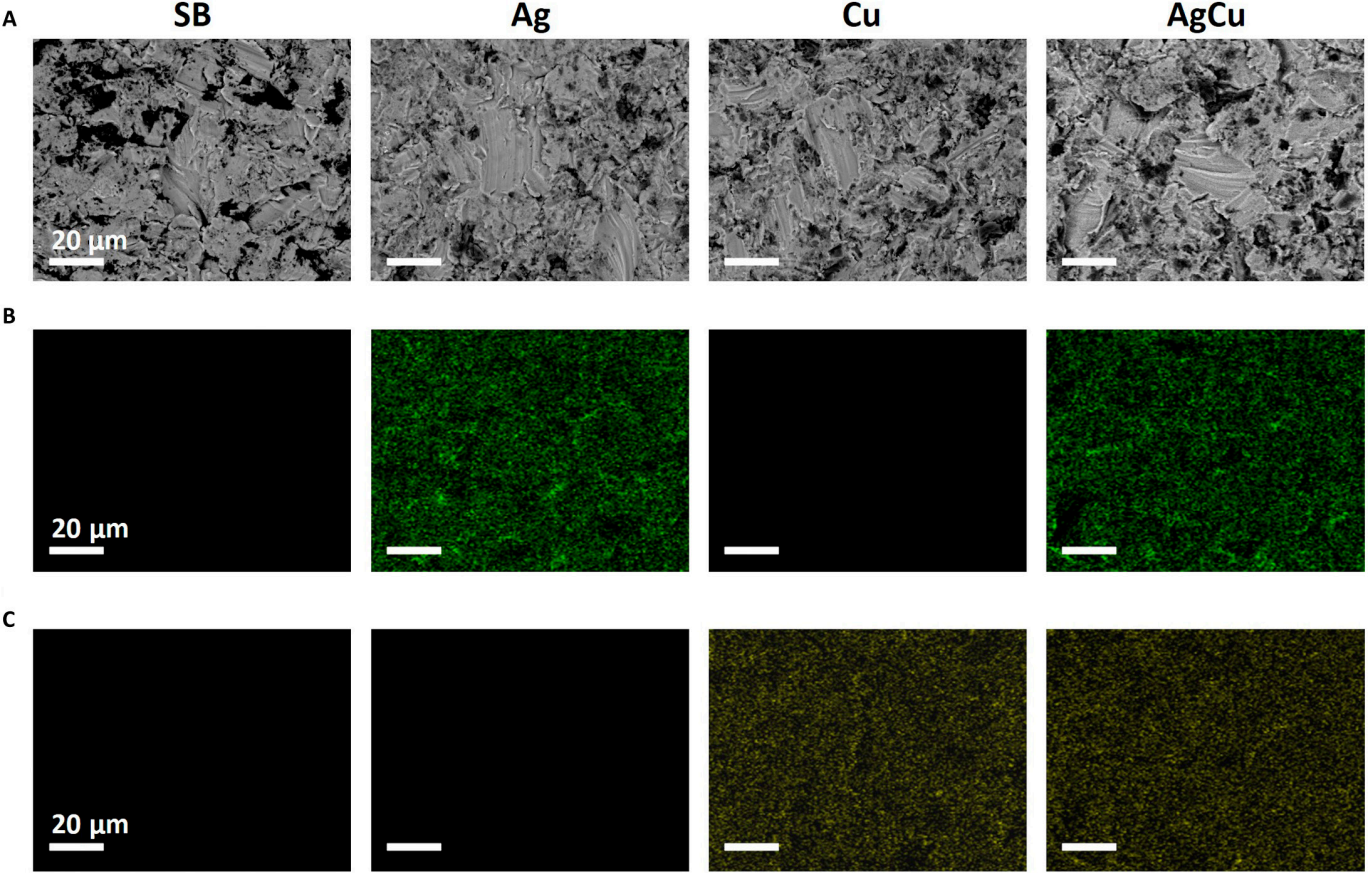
(A) FESEM images of rough Ti6Al4V samples (SB), Ti samples monoimplanted with silver (Ag), Ti samples monoimplanted with copper (Cu), and Ti samples coimplanted with silver and copper (AgCu). (B) Energy-dispersive x-ray (EDX) color mapping of Ag atoms in SB, Ag, Cu, and AgCu samples. (C) EDX color mapping of Cu atoms in SB, Ag, Cu, and AgCu samples. Scale bars = 20 μm.
